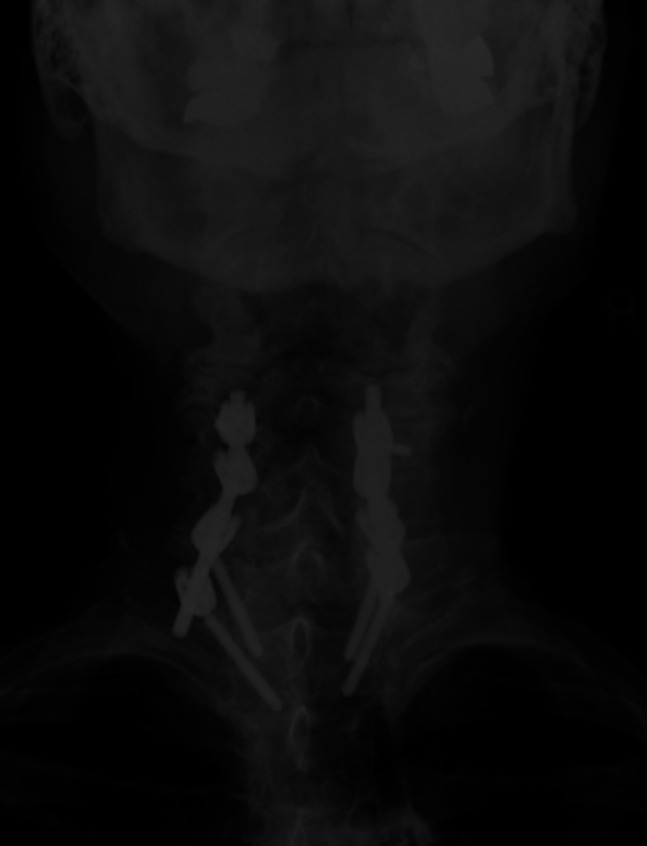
Anterior-posterior radiograph of the patient at 6 months after C5 to T2 posterior cervical fixation.
